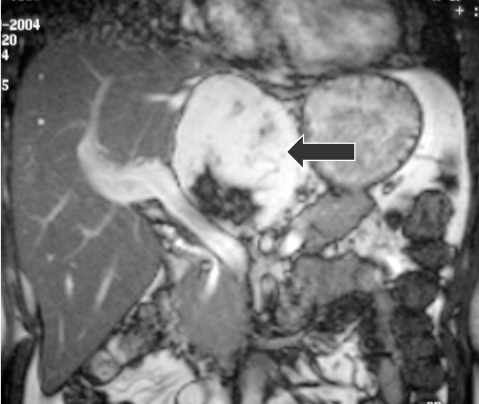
Magnetic Resonance Imaging (MRI) sequence (coronal view). The mixed signal intensity mass (black arrow) appears separate from the portal vein and the arterial branches.
